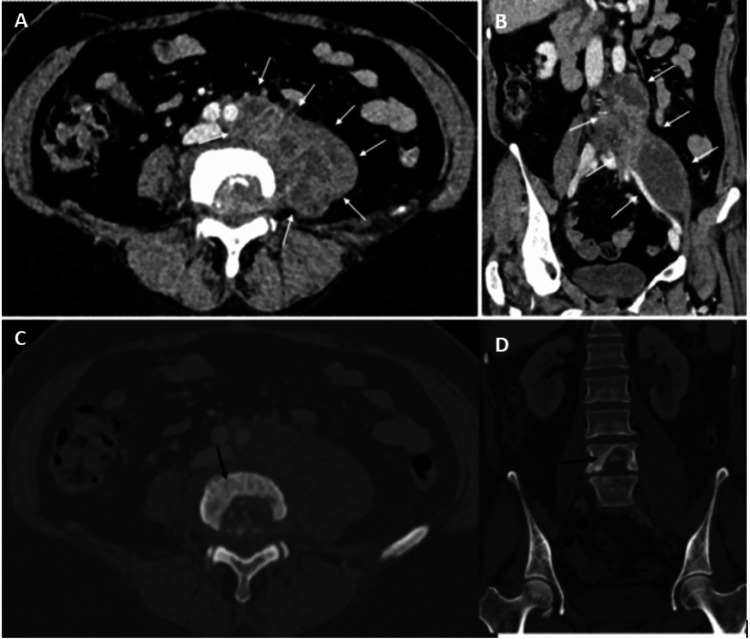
Axial and coronal images Axial (A) and coronal (B) images from a portal venous phase CT scan show a large left psoas abscess (white arrows) and a destructive lesion in the L4 vertebral body (black arrows), which is better appreciated on the bone-window axial (C) and coronal (D) images. CT, Computed Tomography
